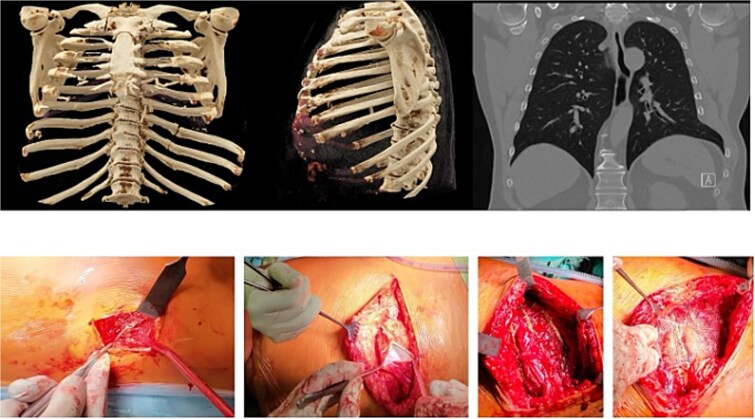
3D-CT reconstruction of the thorax with fractured ribs 8 and 9 on the left posterior column of the chest wall. Also shown in the coronal view of the pre-operative CT scan is shown the costal margin rupture (right upper image) between the seventh and eighth rib anterolateral with a herniation of the lung and the diaphragm. The bottom images show intraoperative images of the preparation of the surgical field: left: debridement of the eighth and ninth rib non-union and bone grafting the gap. Second left: dissection of the lungs herniation, diaphragm, and peritoneum in the anterolateral costal margin rupture. Third left: fixing and reconstruction of the costal margin with using KLS Martin flexible plates with 7 mm angulated screws, as well as applying plates to the unfractured sixth and seventh rib as well as eighth and ninth rib, to prevent the fibre tapes from cutting in the ribs when reconstruction of the intercostal rupture. Right image: applying an additional mesh to securely fix the ribs towards each other and prevent secondary redislocation as well as spreading the force.
